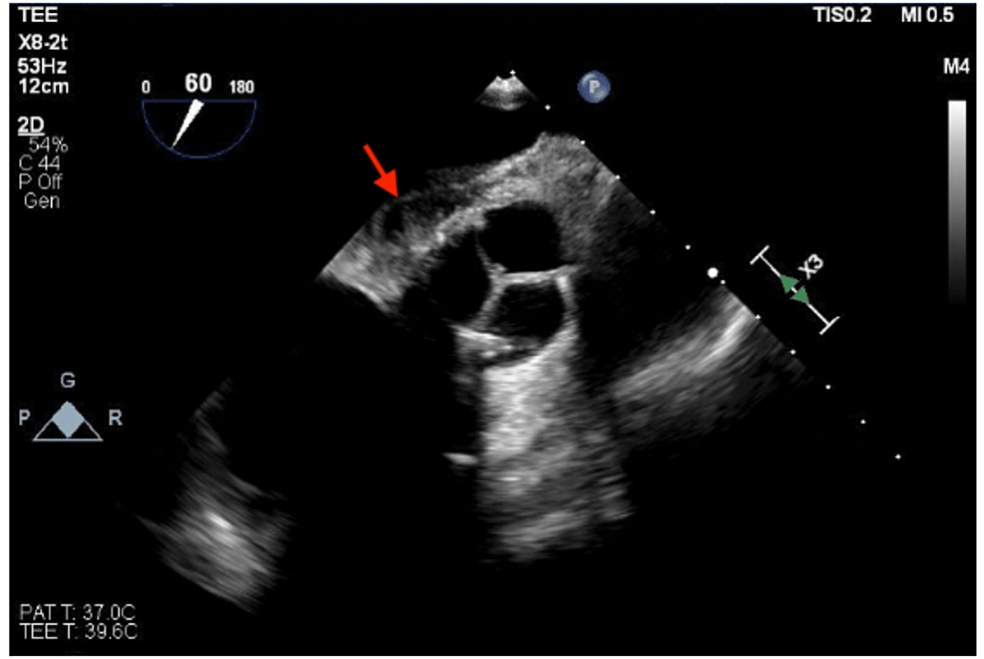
Transthoracic echocardiogram. Transthoracic echocardiogram showed a perivalvular echolucent area between the aortic valve and left atrium concerning an aortic root abscess (red arrow).
